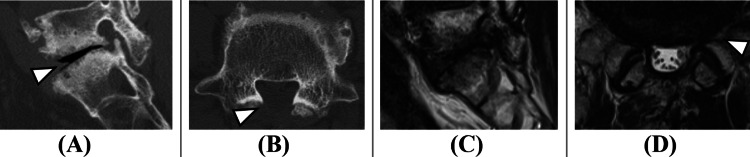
Preoperative images. (A) Sagittal and (B) axial lumbar CT view. (C) Sagittal and (D) axial lumbar MRI view. Following prior lumbar disc herniation surgery, partial resection of the L5 lamina is visible (white arrowhead, B). Disc space collapse, suggestive of segmental instability, is noted (white arrowhead, A). Left L5 foraminal stenosis is also evident (white arrowhead, D), indicating likely compression of the left L5 nerve root and resulting in sciatica symptoms.
